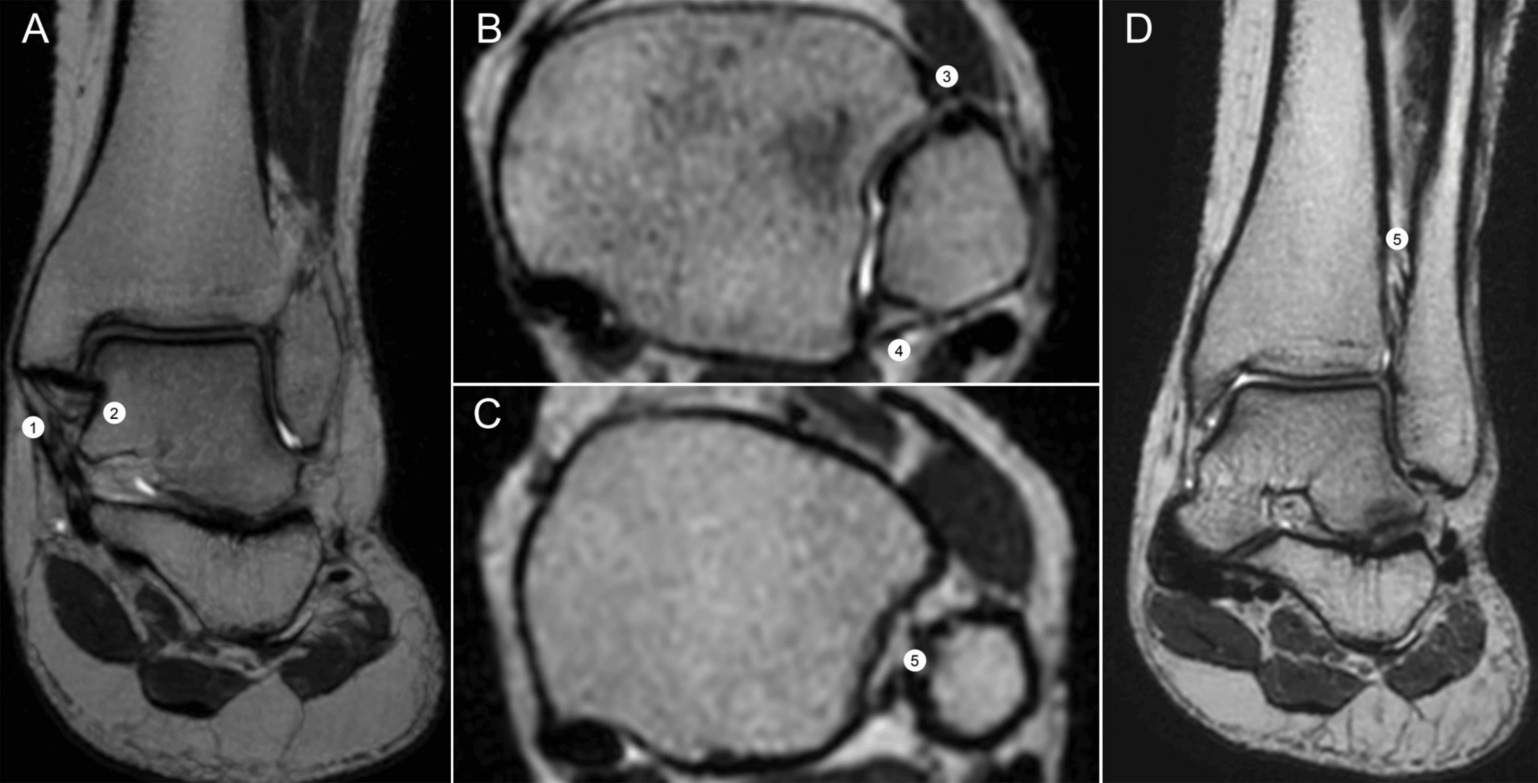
Medial stabilizers and syndesmotic ligaments on CUBE MRI. Three-dimensional T2-weighted reconstructions show (A) the deltoid ligament with superficial fibers (1) and deep fibers (2); (B) anterior (3) and posterior (4) tibiofibular syndesmotic ligaments; (C, D) the interosseous ligament (5). Images belong to the authors; patient consent was obtained.
